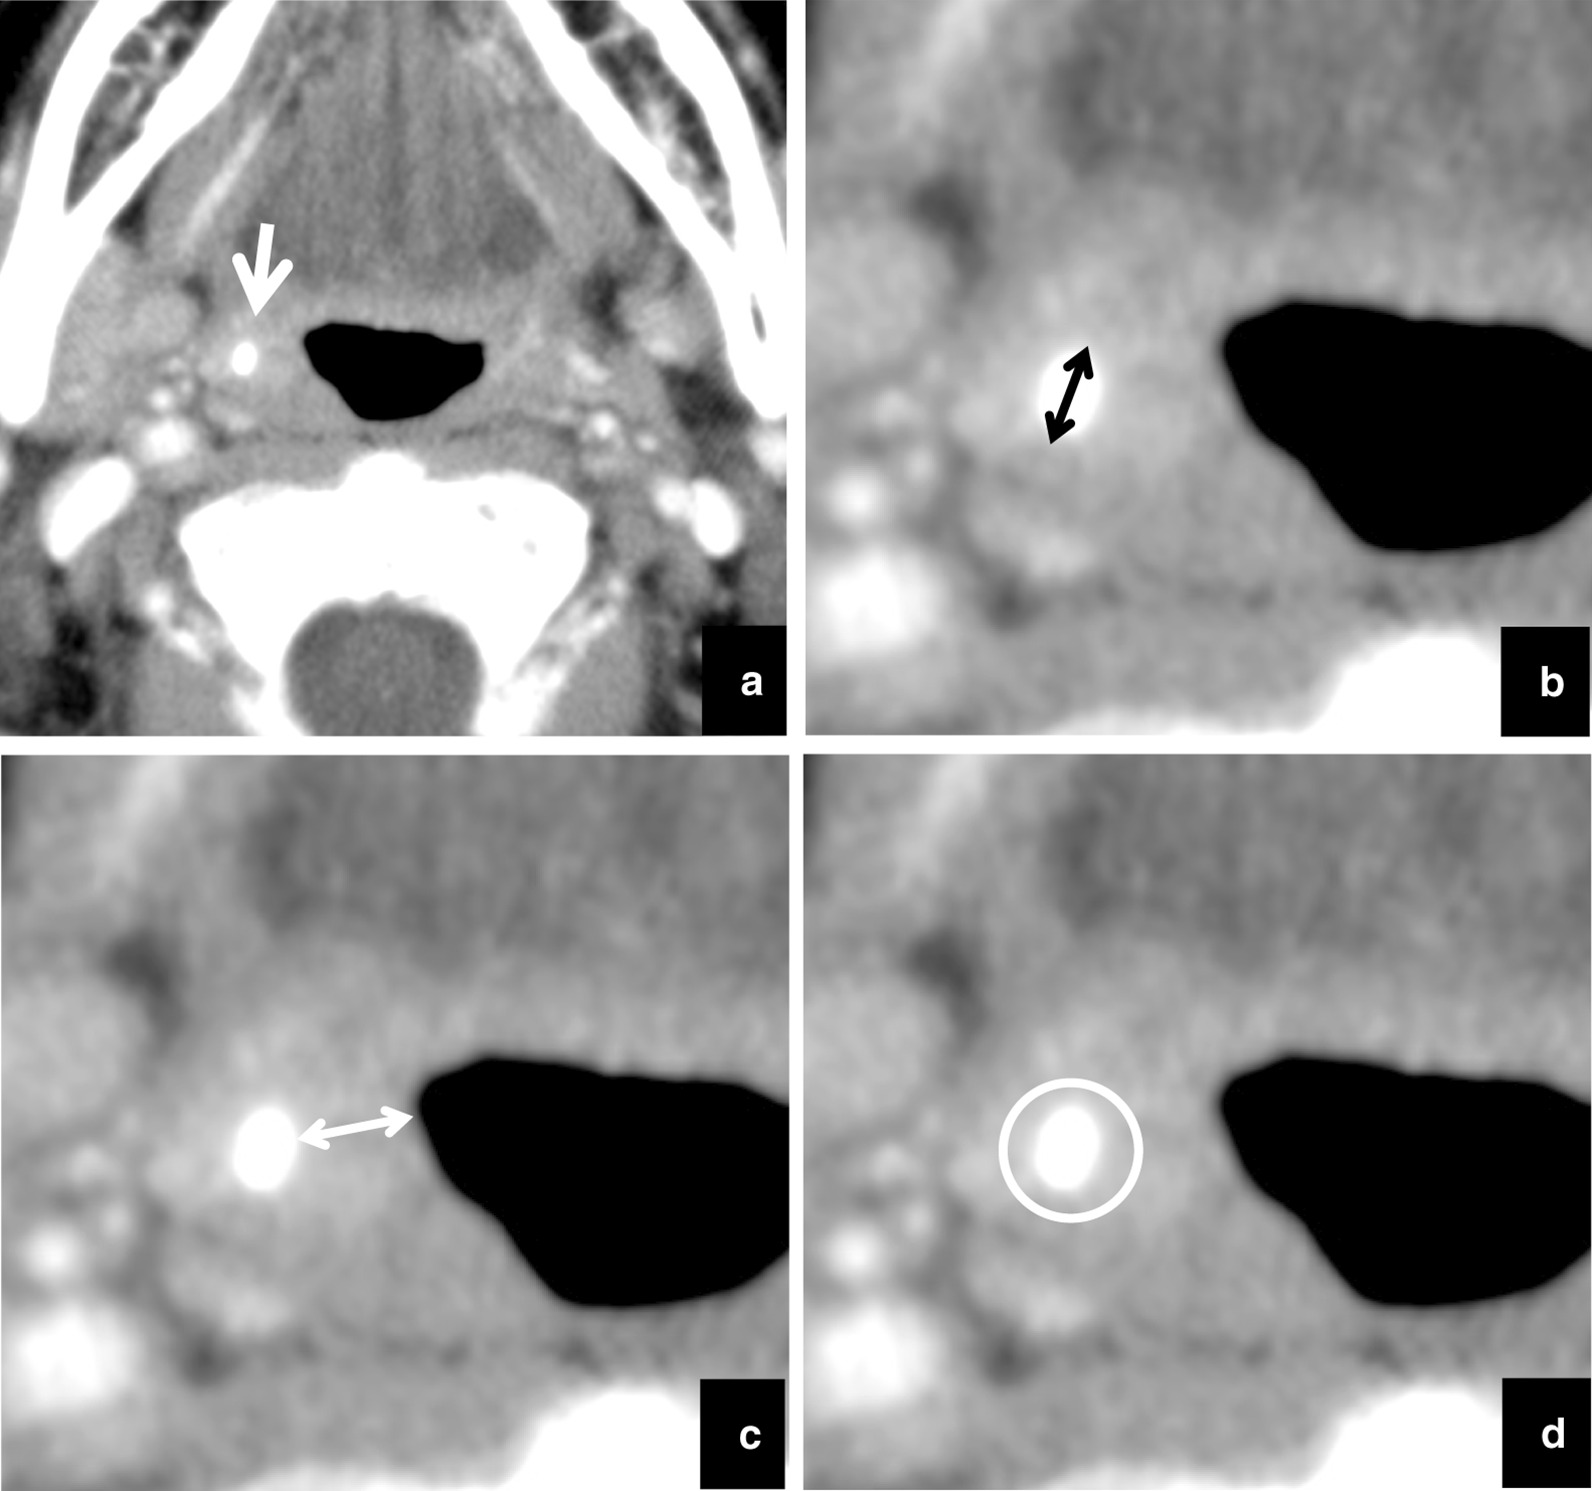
Methods for measurement of size, CT number, and depth of tonsilloliths on CT images. a The largest calculus (soft-tissue window setting, arrow) in the palatine tonsil was included in the analysis. b Size was defined as the long axis measurement (arrow) on soft-tissue window setting. c Position was defined as the shortest distance (arrow) from the surface of the respiratory tract on soft-tissue window setting. d Calcification level was defined as the maximum HU number on soft-tissue window setting, and the region of interest included the whole calculus (circle)
